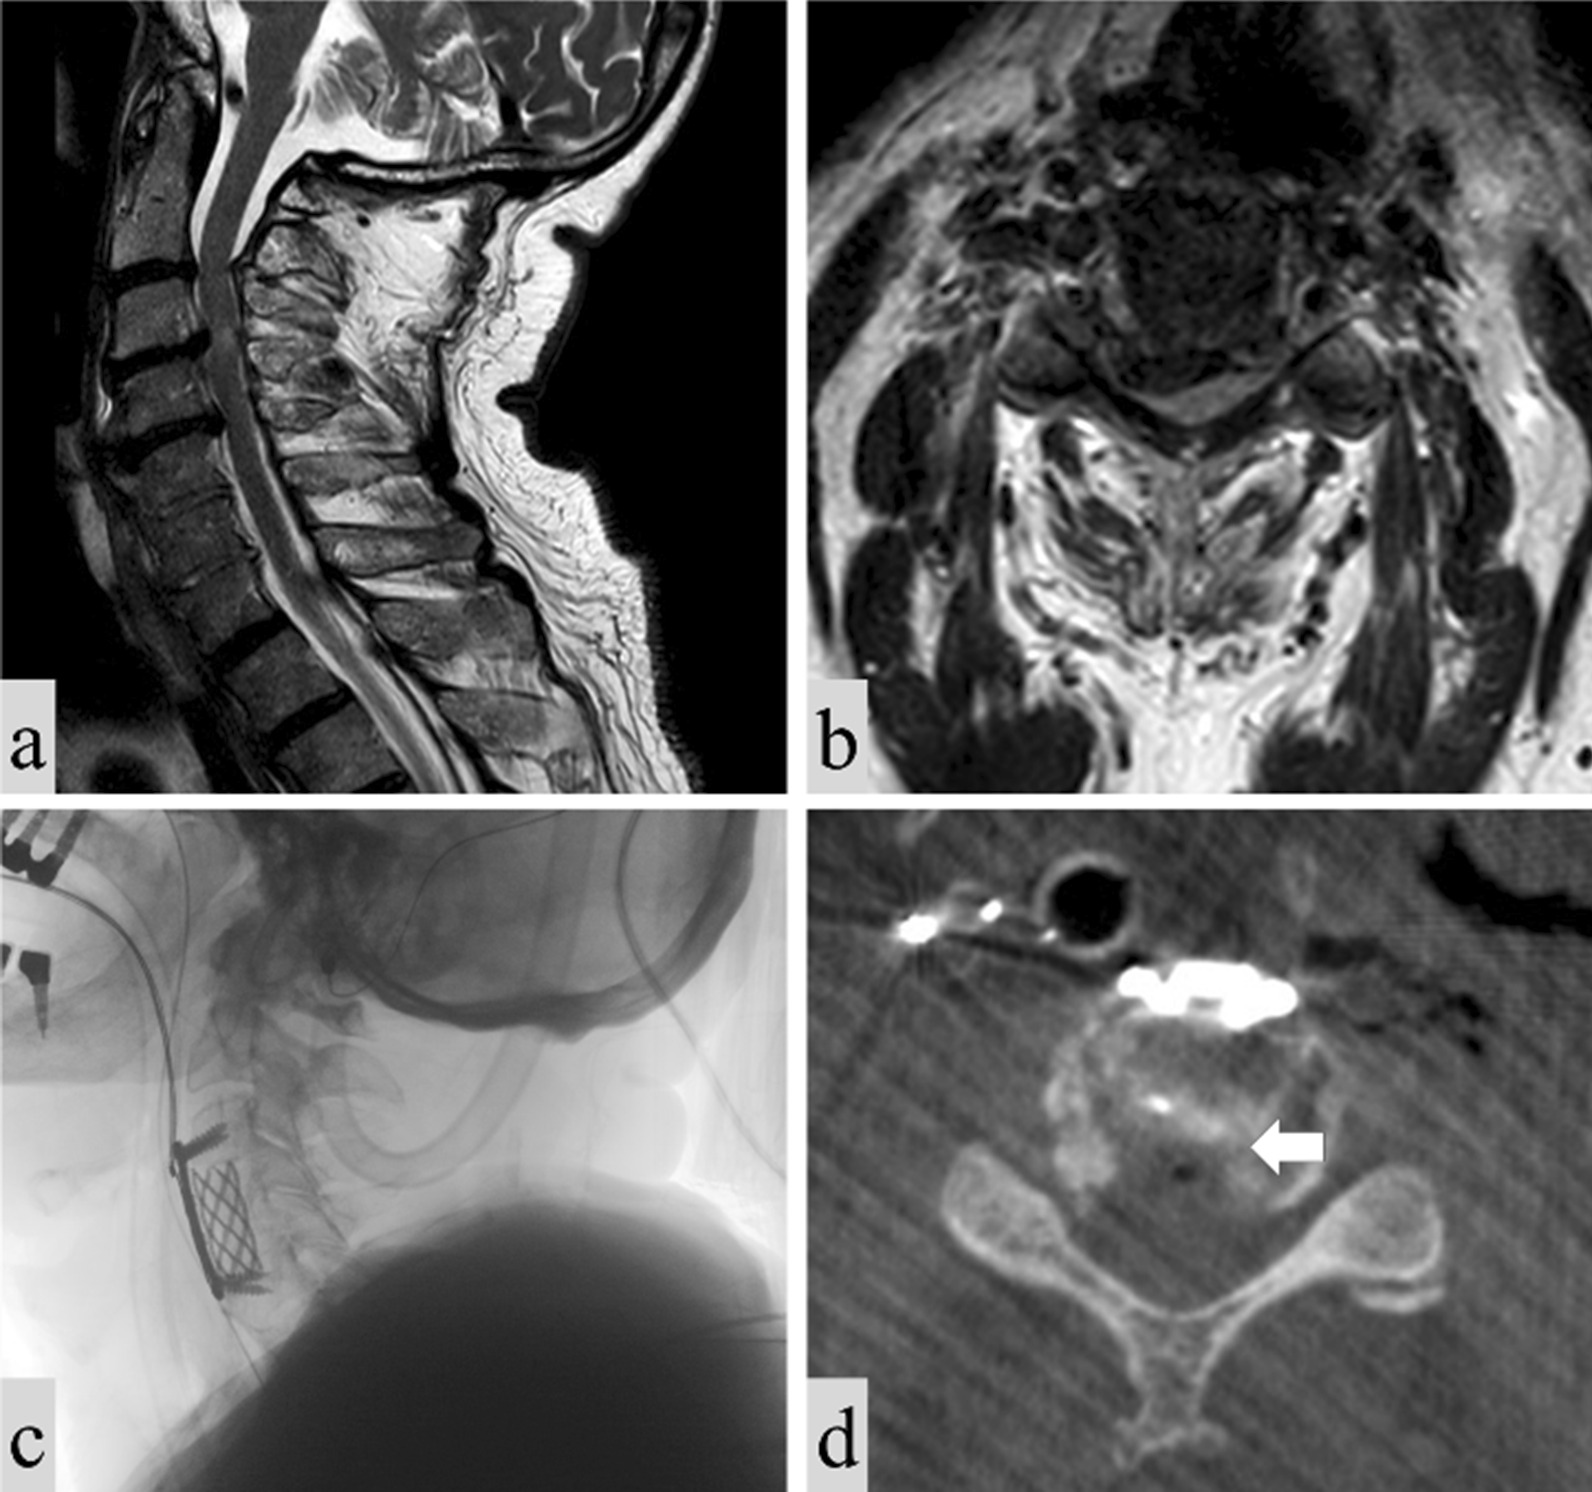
Imaging scans of a patient treated for C3–C5 stenosis. a Preoperative MRI, sagittal view; b preoperative MRI, transverse view; c intraoperative x-ray, sagittal view; d intraoperative CT scan, axial view of the treated region, demonstrating successful removal of the osteophyte (marked by an arrow) without bone removal at the vertebral posterior side or compromising the integrity of the adjacent vertebrae
